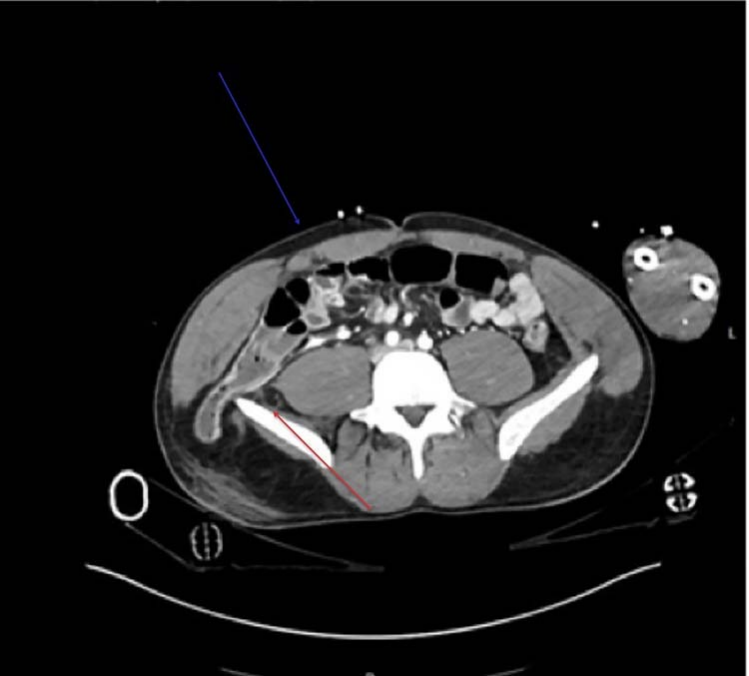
Axial view of the CT demonstrates the tear in the rectus muscle (blue arrow). Inferior lumbar hernia can be clearly demonstrated, above the iliac crest (red arrow).
